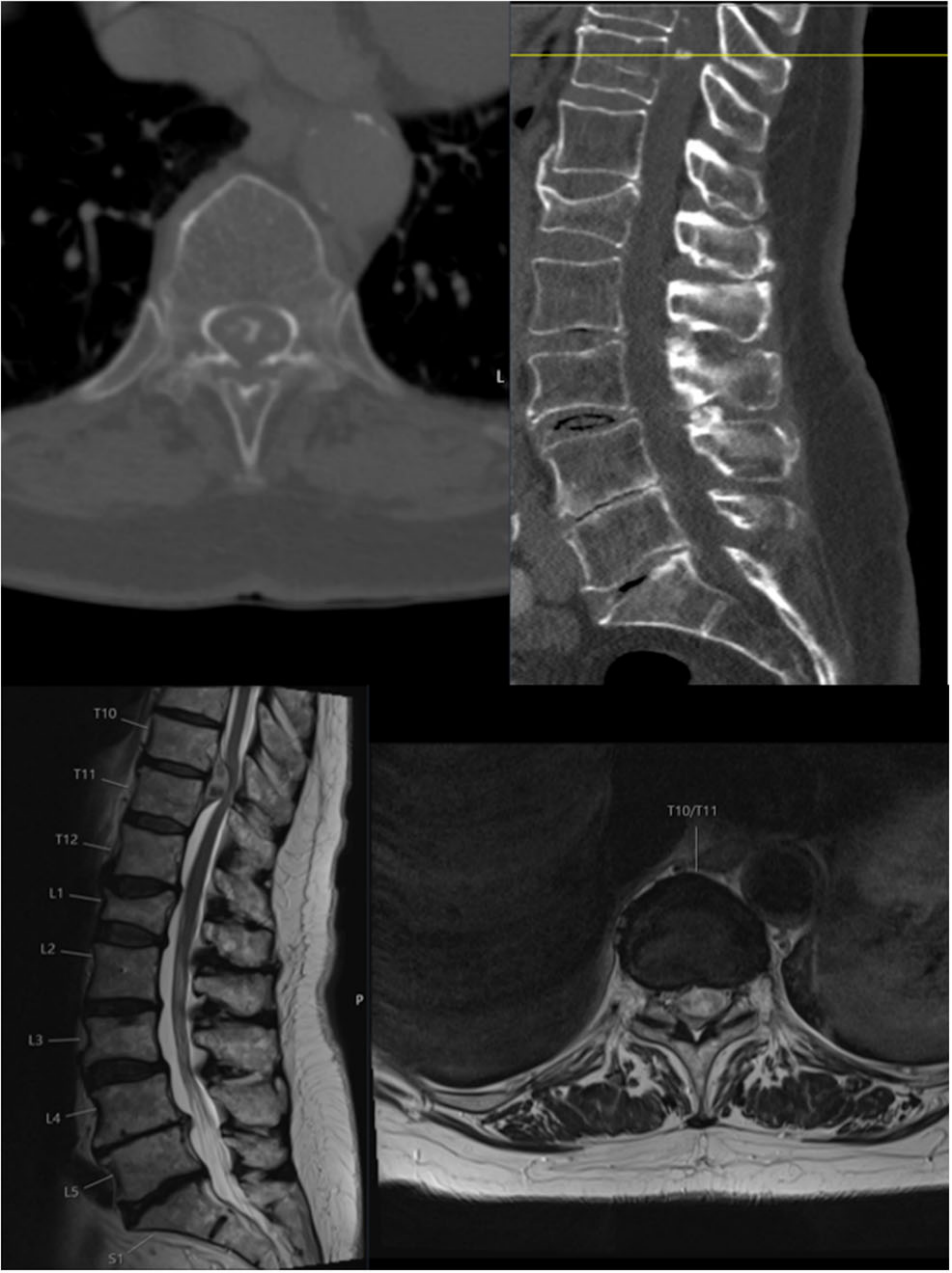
Preoperative CT (upper image) and MRI (lower image) scan of a patient suffering from a calcified giant disc herniation at the level Th10/11 with axial and sagittal plane showing the calcification (➔) and compression of the spinal cord
